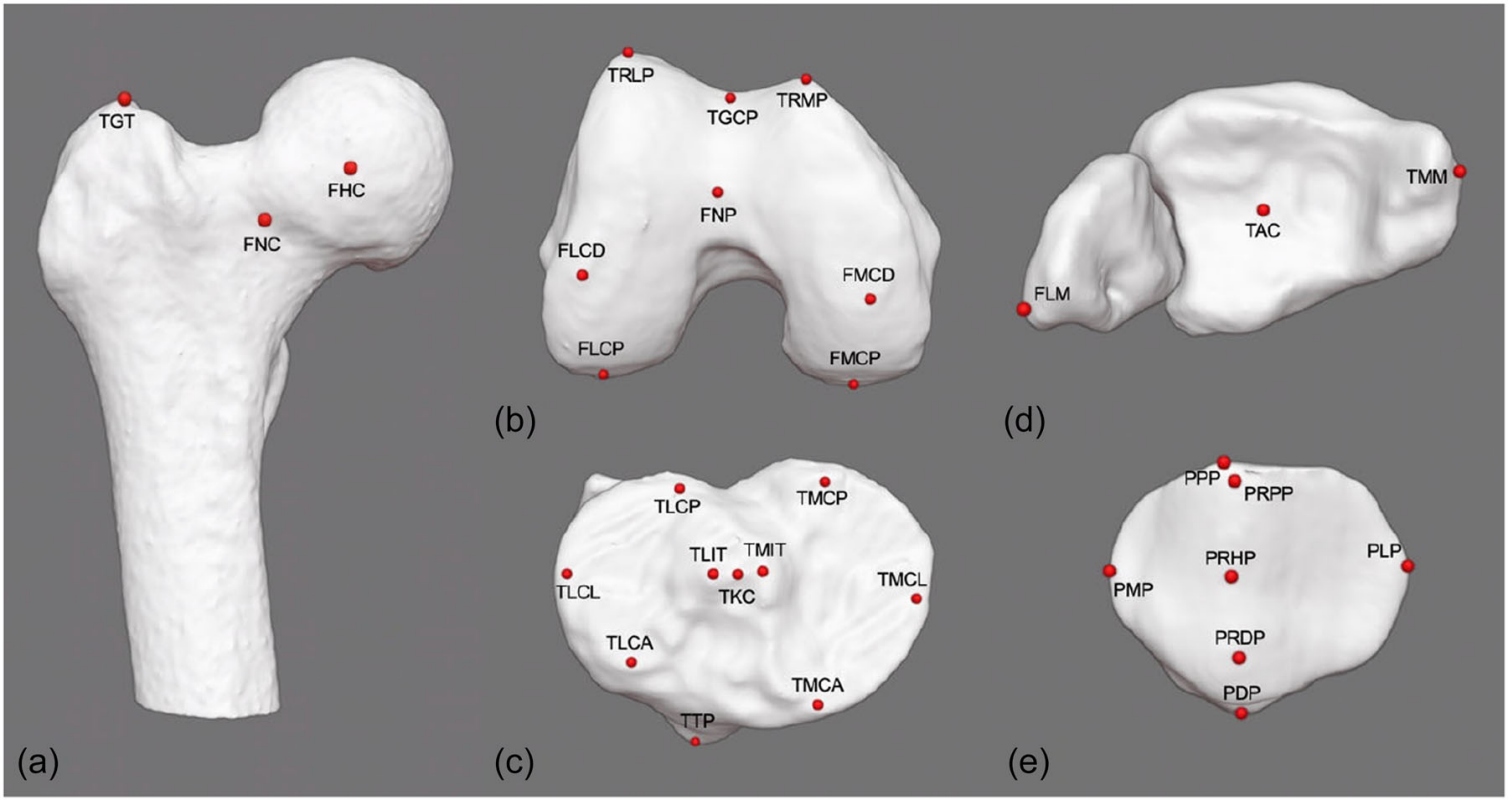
3D reconstruction by CBCT of (a) proximal femur, (b) distal femur, (c) proximal tibia, (d) ankle and (e) patella showing all 31 bony landmarks. CBCT, cone bean computed tomography; FHC, femoral head center; FLCD, femoral lateral condyle distal; FLCP, femoral lateral condyle posterior; FLM, fibular lateral malleolus; FMCD, femoral medial condyle distal; FMCP, femoral medial condyle posterior; FNC, femoral neck center; FNP, femoral notch point; PDP, patella distal pole; PLP, patella lateral pole; PMP, patella medial pole; PPP, patella proximal pole; PRDP, patella ridge distal pole; PRHP, patella ridge high point; PRPP, patella ridge proximal pole; TAC, tibial ankle center; TGT, tip of greater trochanter; TKC, tibial knee center; TLCA, tibial lateral condyle anterior; TLCL, tibial lateral condyle lateral; TLCP, tibial lateral condyle posterior; TLIT, tibial lateral intercondylar tubercle; TMIT, tibial medial intercondylar tubercle; TMCA, tibial medial condyle anterior; TMCM, tibial medial condyle medial; TMCP, tibial medial condyle posterior; TMM, tibial medial malleolus; TGCP, trochlear groove central point; TRLP, trochlear ridge lateral point; TRMP, trochlear ridge medial point; TTP, tibial tuberosity point.
